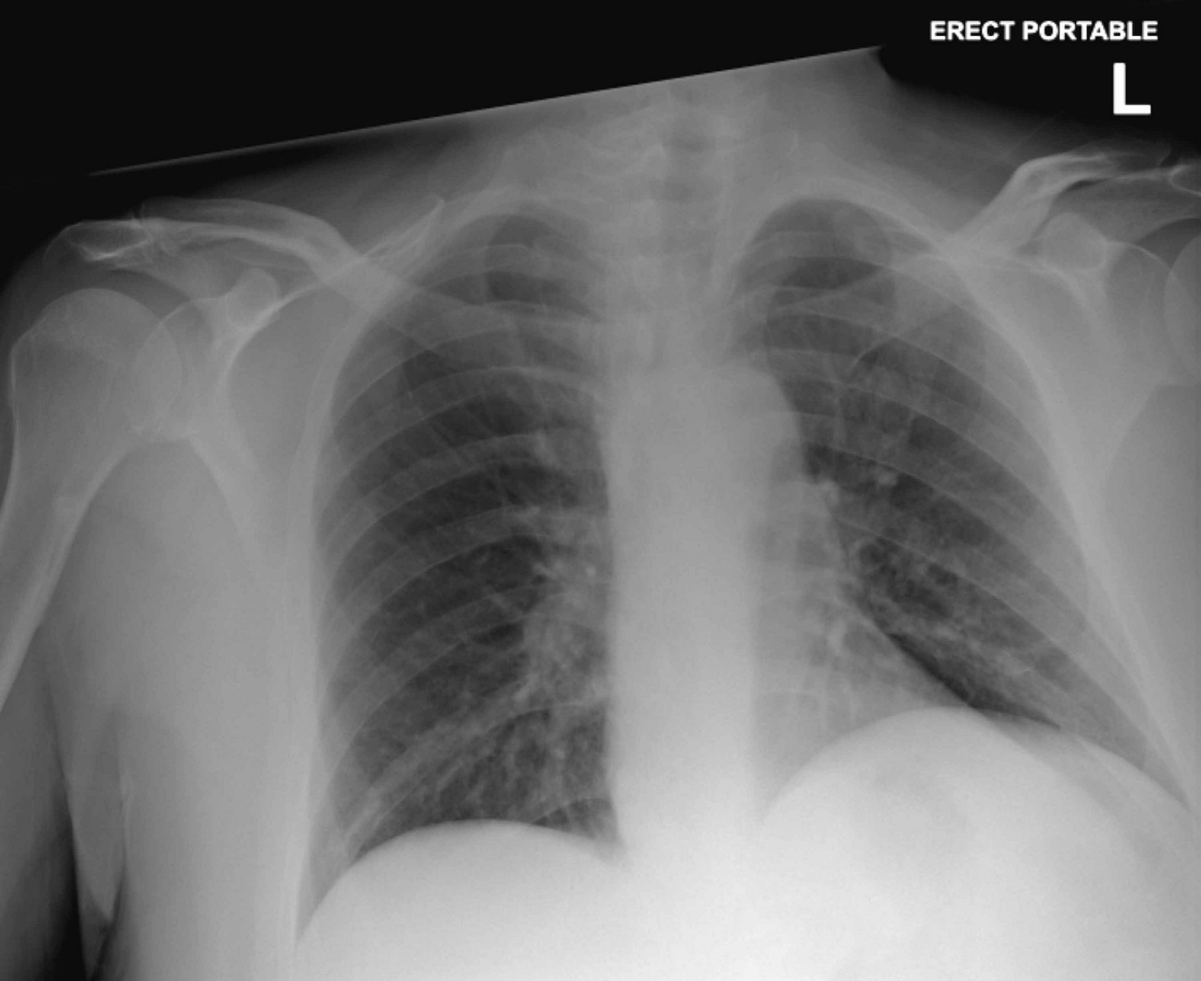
A single anteroposterior view of the chest via chest x-ray No acute disease is displayed. There are signs of degenerative changes in the shoulders.
